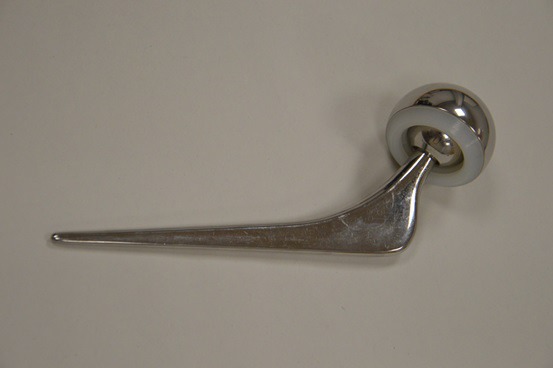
Radiograph of a cemented hemiarthroplasty with a unipolar articulation demonstrating centre edge angle (yellow) and residual femoral neck length (green).
